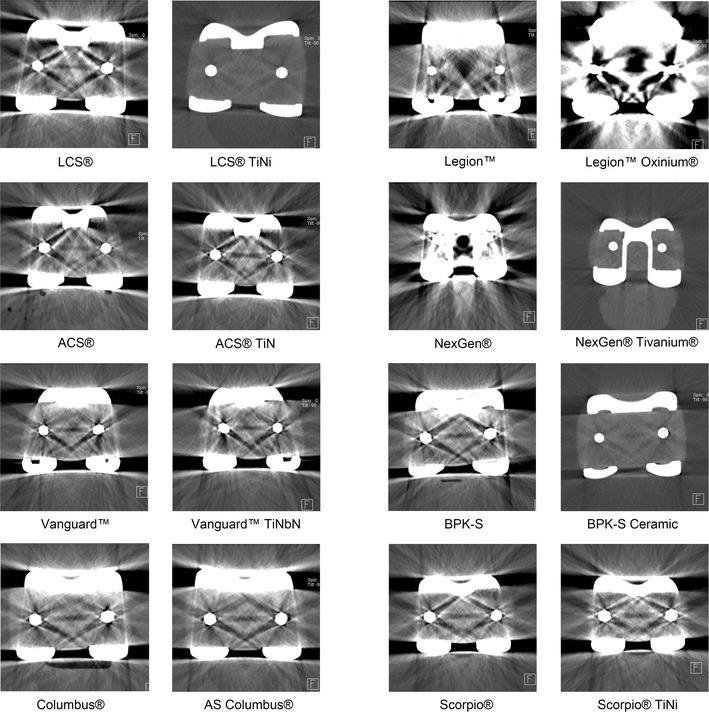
CT images of all vendor prostheses pairs showing the extent of the artifacts. For all images in this gallery, scan protocol 7 (dual-energy CT with mono-energetic imaging at 105 keV) was used. It provided the lowest amount of streak artifacts of all protocols
